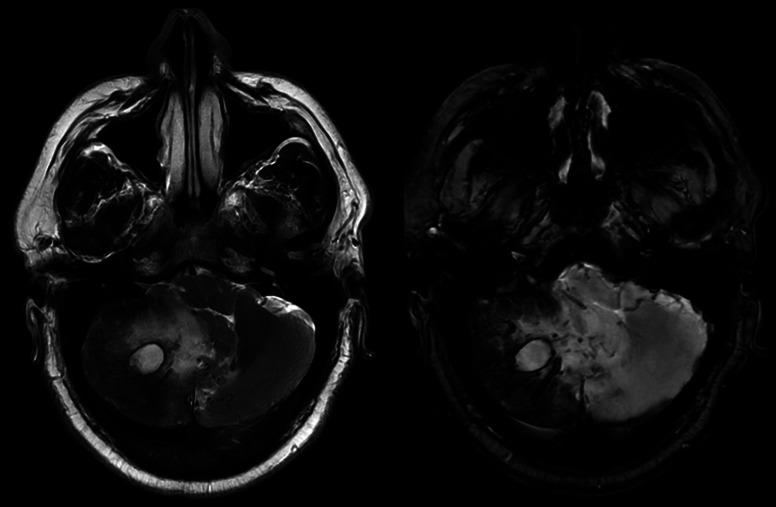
Axial magnetic resonance imaging of the cerebellum with T2-weighted images (left) and T2*-weighted images (right). On the left image, notice the edema surrounding the lesion in the right cerebellum with mass effect on the fourth ventricle. Visualization of hemosiderin (dark rim) due to prior hemorrhage on both, although more pronounced on the T2* sequence.
